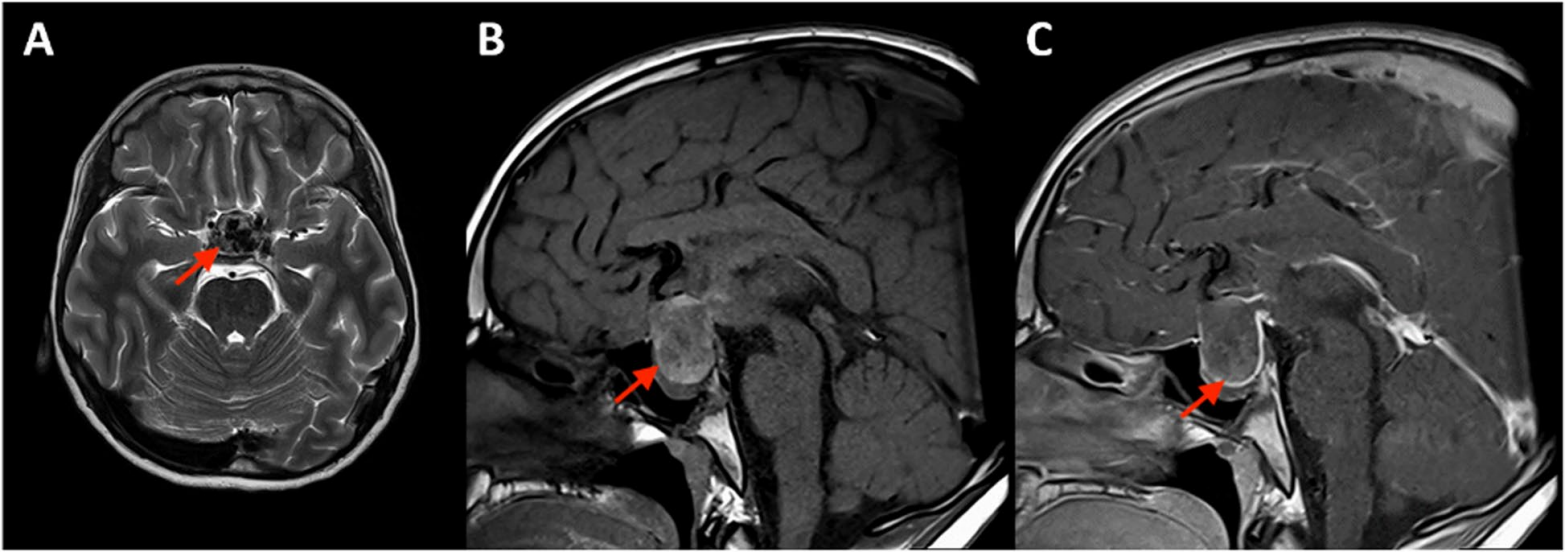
Cerebral MRI showing a cystic tumor in the intra- and suprasellar region, hypointense in the axial T2-weighted image (A), spontaneously hyperintense in the sagittal T1-weighted image (B) with an enhancement of the cyst wall after gadolinium injection (C)
